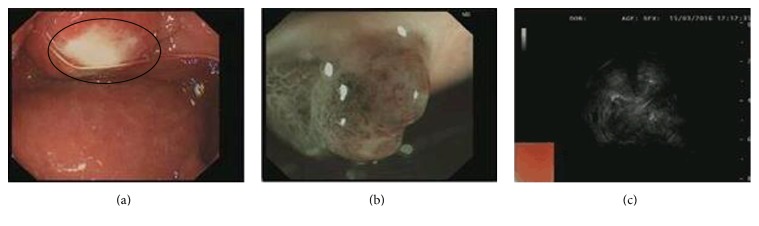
Electronic gastroscope and endoscopic ultrasonography both showed the abnormality of the duodenal mucosa. (a) Electronic gastroscope revealed that there was a mucosal protrusion at the first portion of the duodenum (duodenal bulb) with an ulcerated surface (marked with a circle). (b) NBI and ME showed that the local vascular was disordered, and gland duct disappeared. (c) EUS showed that the hypoechoic mass with uniform echo was at duodenal bulb.
